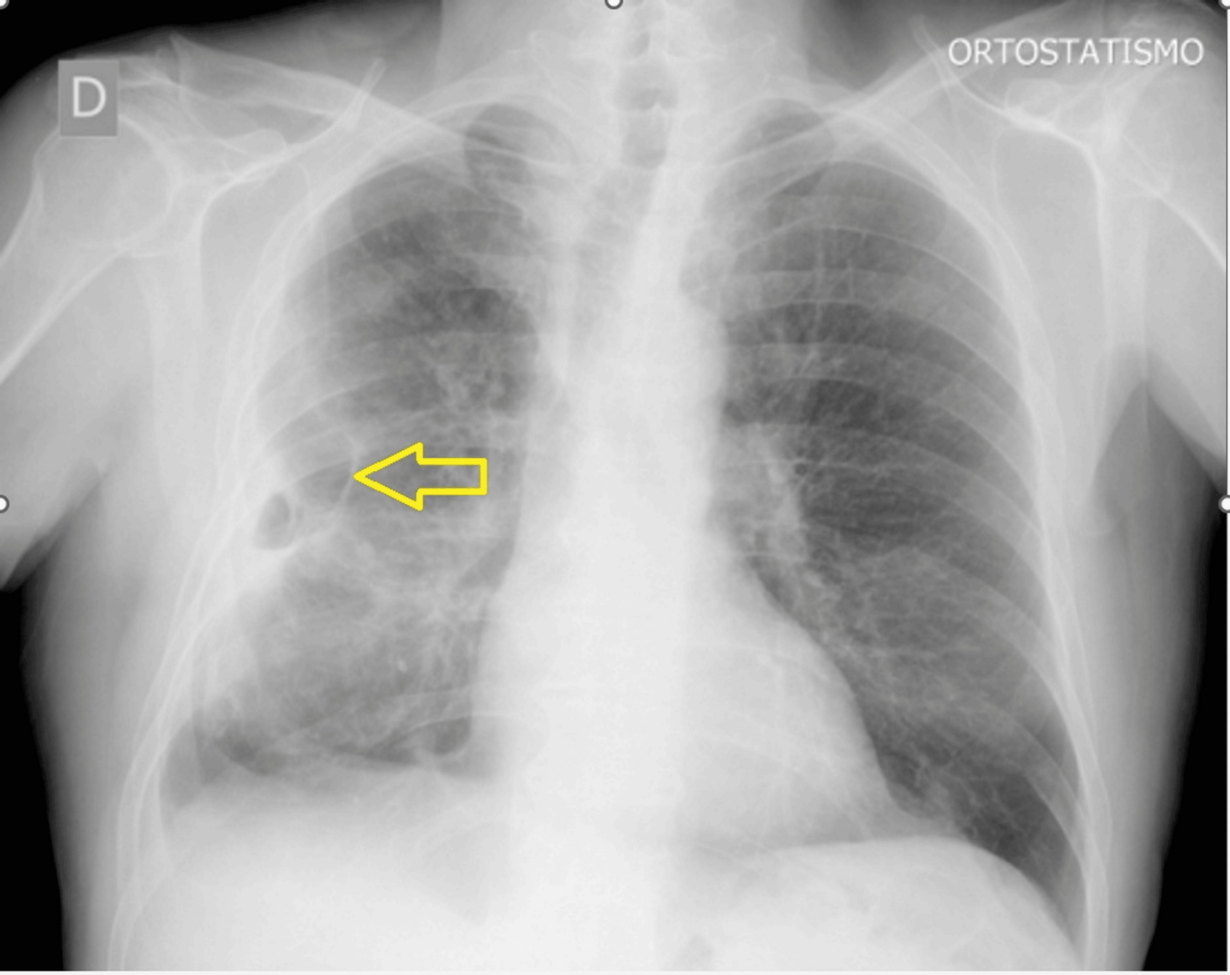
Chest X-ray in posteroanterior incidence The image shows nodular image on the right lung (yellow arrow), which might have been infectious etiology or neoplasm. We also identified a slight effacement of costophrenic sinuses, which was suspected to be a pleural effusion. No others changes were noted.
